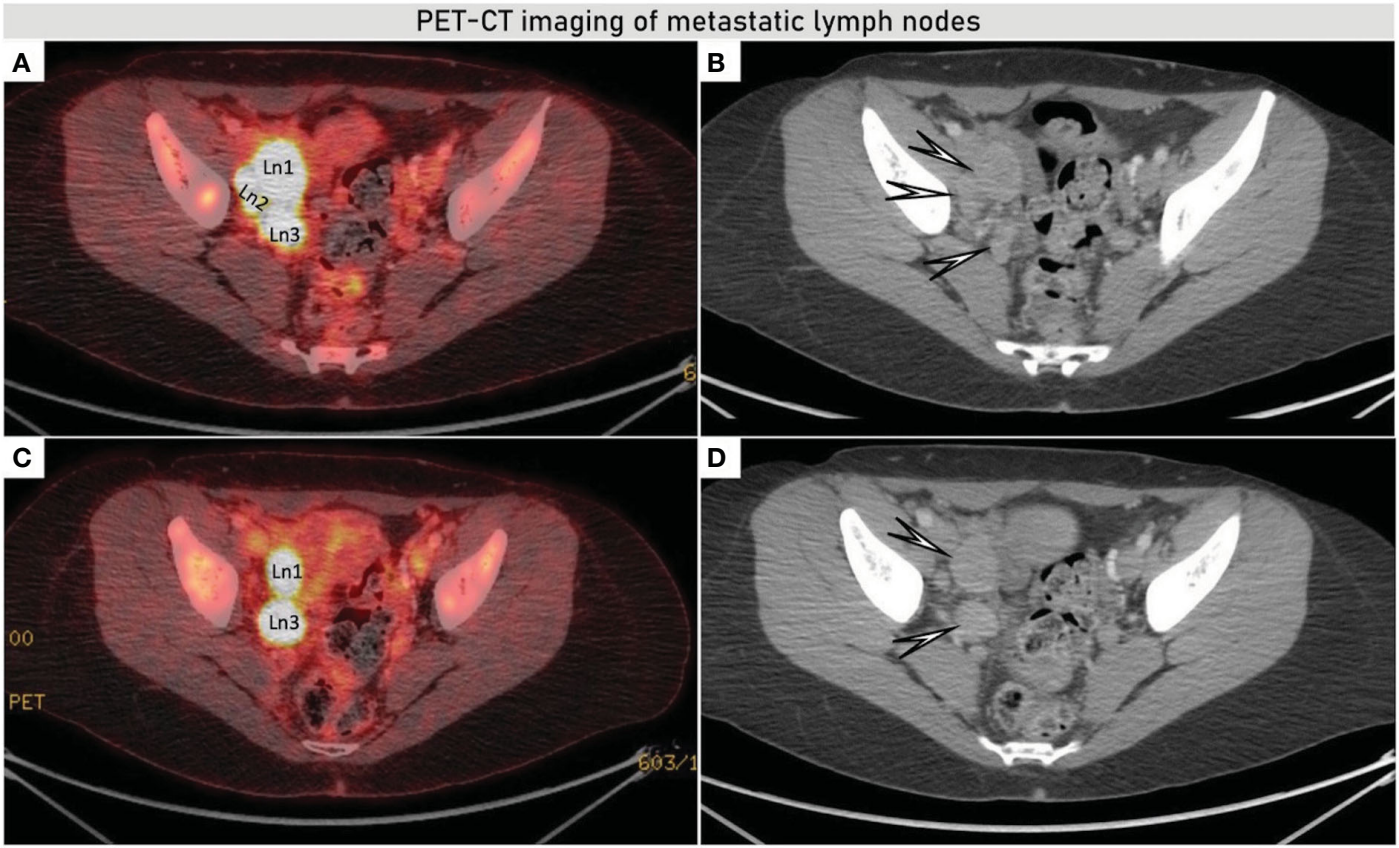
Axial fused PET-CT showed increased accumulation of 18F-FDG in the bulky iliac lymph nodes on the right obturator fossa of up to 36 × 25 mm (A, C), morphologic images from CECT demonstrating enlarged nodes in the right obturator fossa (white arrows) (B, D). PET-CT (positron emission tomography combined with computed tomography), CECT (contrast enhanced computed tomography), and 18F-FDG (18F- fluorodeoxyglucose).
